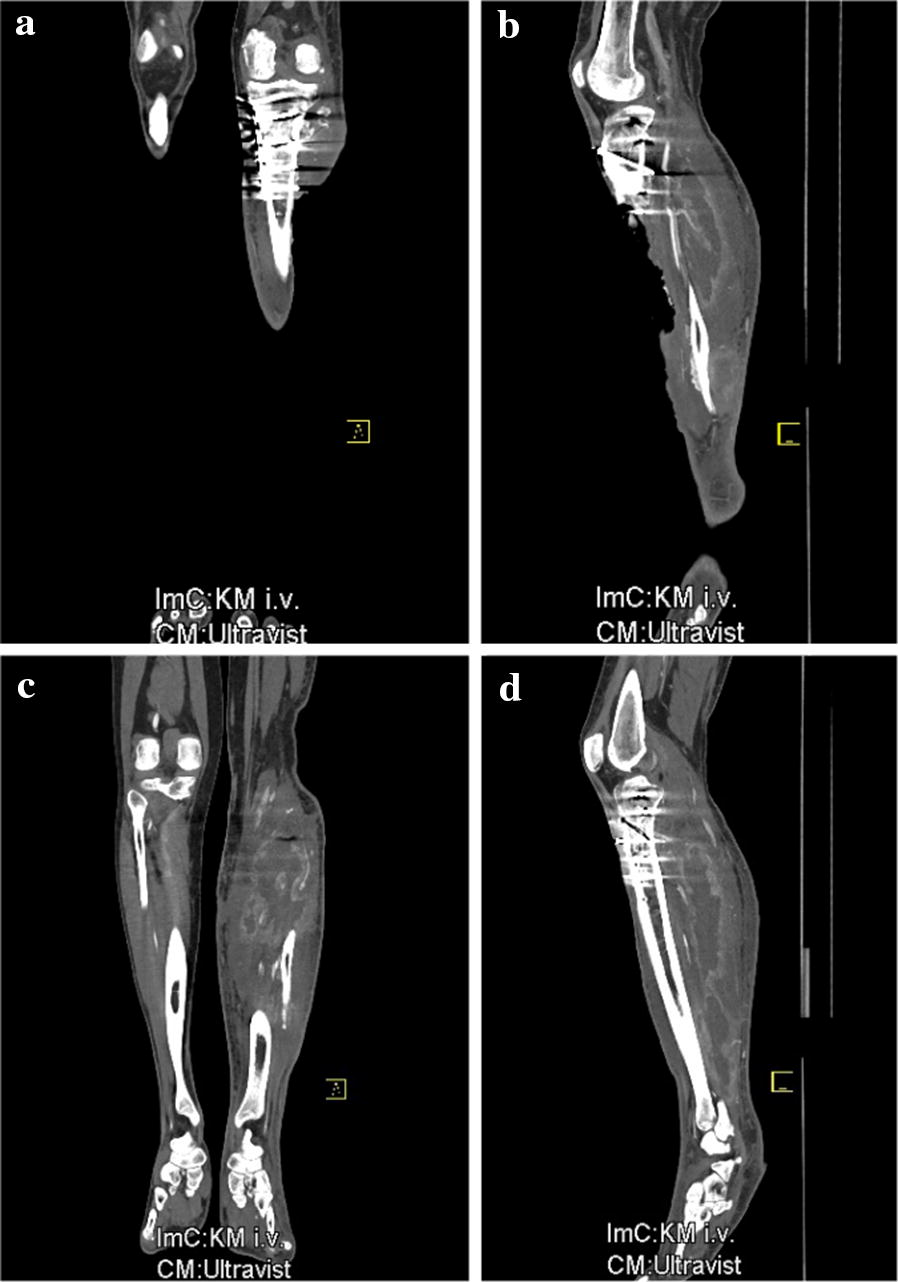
CAT scan at first admission showing severe soft tissue defect zone and abscess formation in the dorsal lower limb muscles. a CT coronary plane showing large lateral defect zone, b sagittal plane with large lateroventral defect zone, c CT coronary plane showing large abscess in the dorsal soft tissue and d sagittal plane showing large dorsal abscess zone
